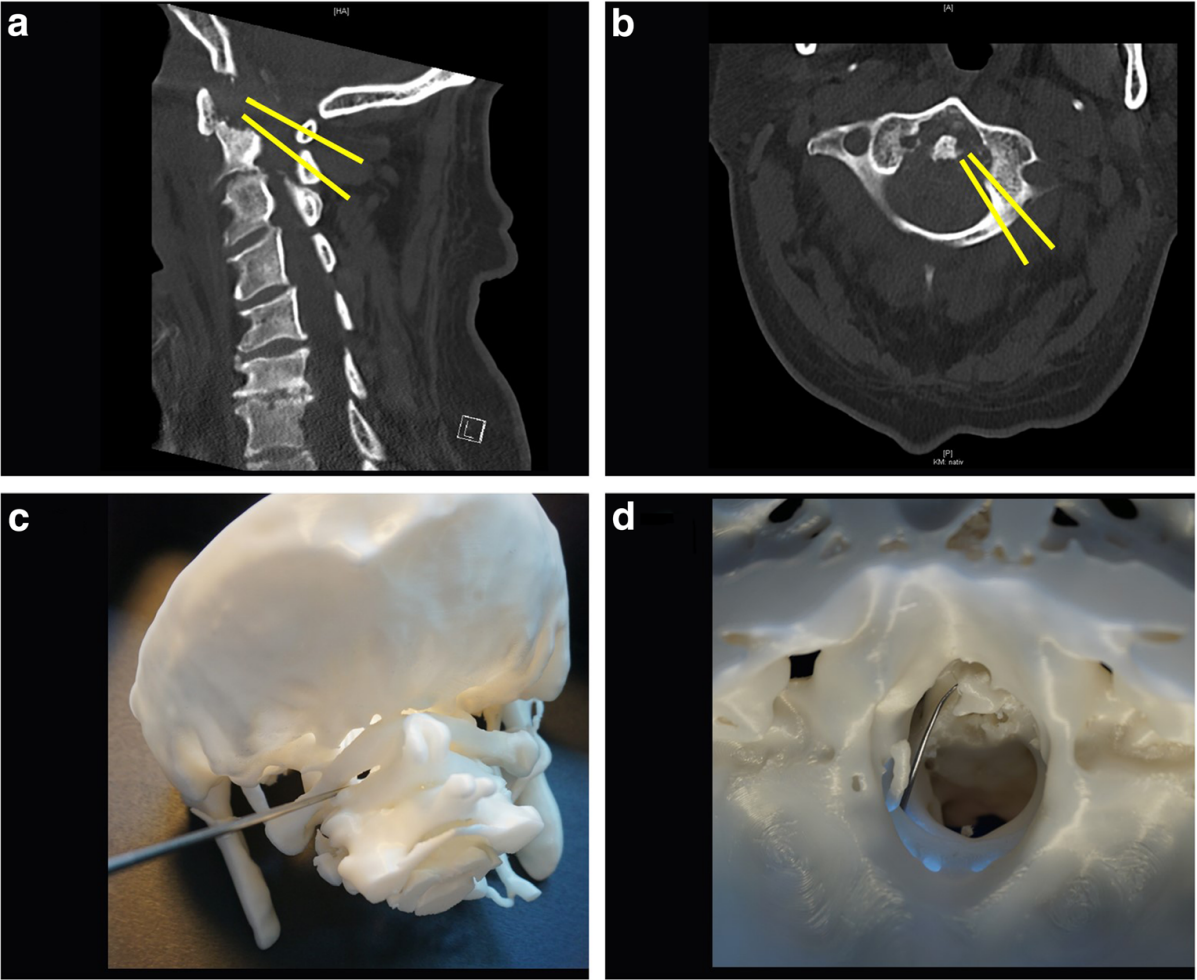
a Sagittal CT (bone window): yellow lines = trajectory of PESCA. b Axial CT (bone window): yellow line = trajectory of PESCA. c 3-Dimensional printing of the cervical spine (the inserted dissector demonstrates the entry point). d 3-Dimensional printing of the cervical spine: view of OP and foramen magnum (the inserted dissector demonstrates that PESCA is possible without relevant traction of the spinal cord)
